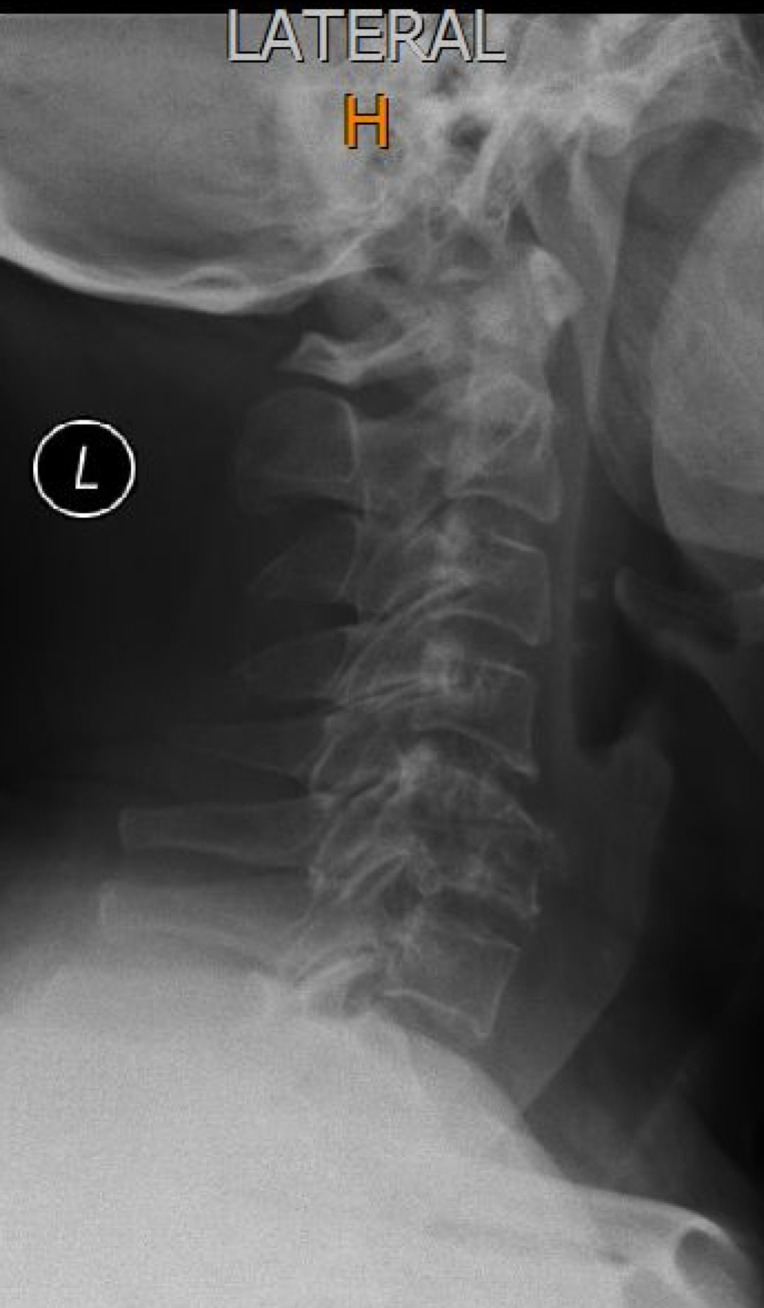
Lateral cervical spine radiograph showing partial collapse of the C5 and C6 vertebral bodies with complete loss of the C5/6 disc space
